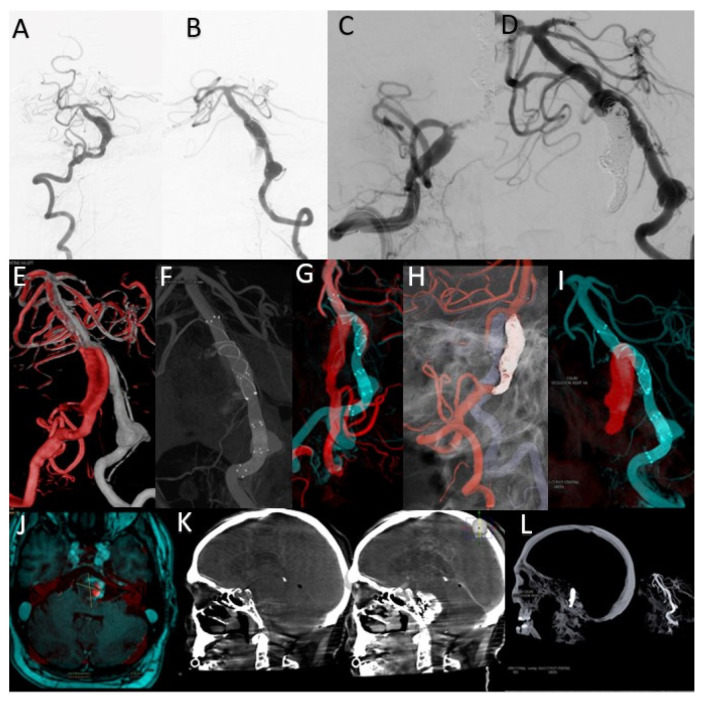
(A) Right VA preoperative DSA. (B) Left VA preoperative DSA. (C) Right VA postoperative DSA. (D) Left VA postoperative DSA. (E) Overlay between the right VA 3DRA (red) and left VA 3DRA (grey). (F) Left VA VasoCT MIP after stents deployment. (G) MIP Overlay between right VA 3DRA (red) and right VA VasoCT (blue) after stents deployment. (H) 3D Roadmap overlay between right VA 3DRA (red) and left VA 3DRA (grey) during the right VA distal segment occlusion. (I) MIP Overlay between the right VA coiled segment 3DRA (red) and right VA VasoCT (blue). (J) Overlay between the 1-month control MRI and left VA VasoCT at the end of the treatment. (K) Overlay between the XperCT preoperative sagittal volume and the XperCT postoperative sagittal volume. (L) Overlay between the XperCT postoperative sagittal volume and the pre-operative left VA 3DRA volume.
